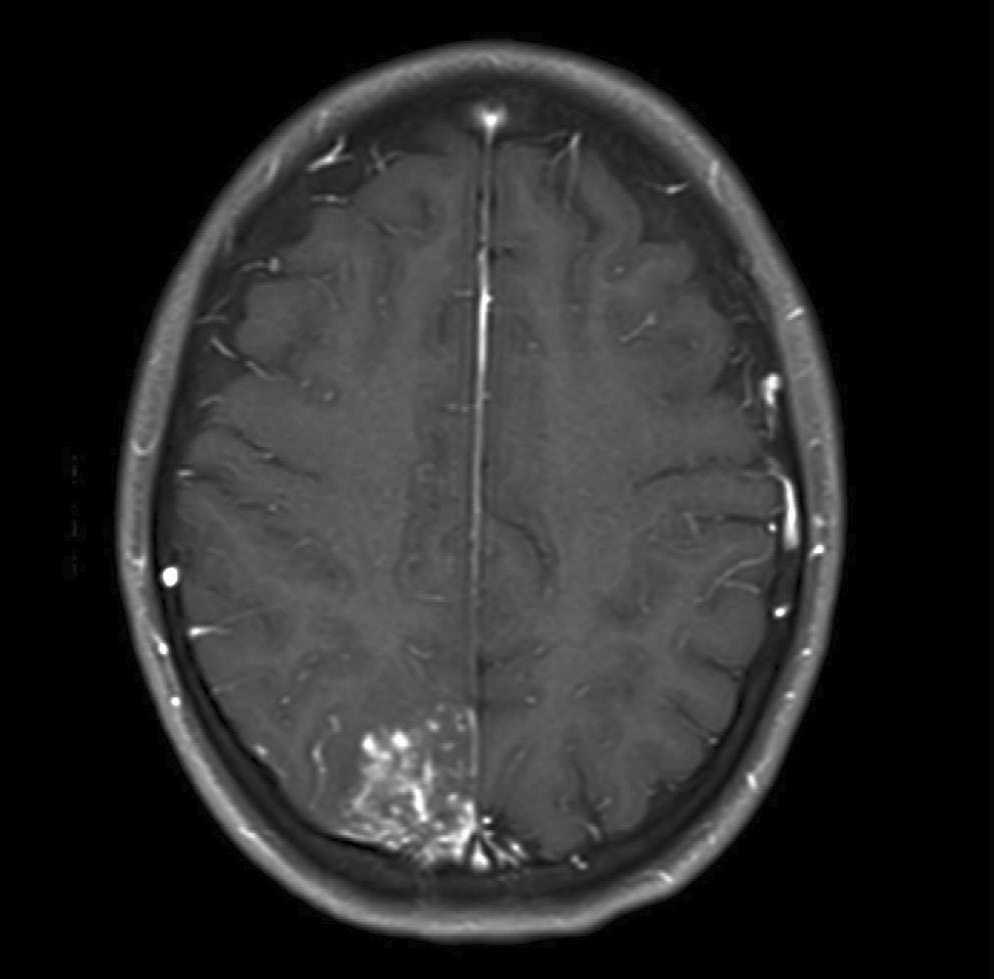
FIGURE 3 Brain MRI in axial T1‐weighted post‐contrast
